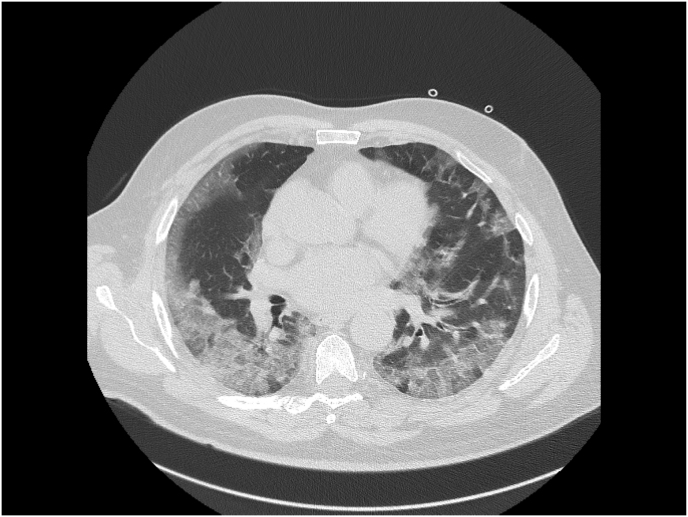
CT scan of the thorax at hospital admission (day 0) demonstrates diffuse subpleural ground-glass opacity.
